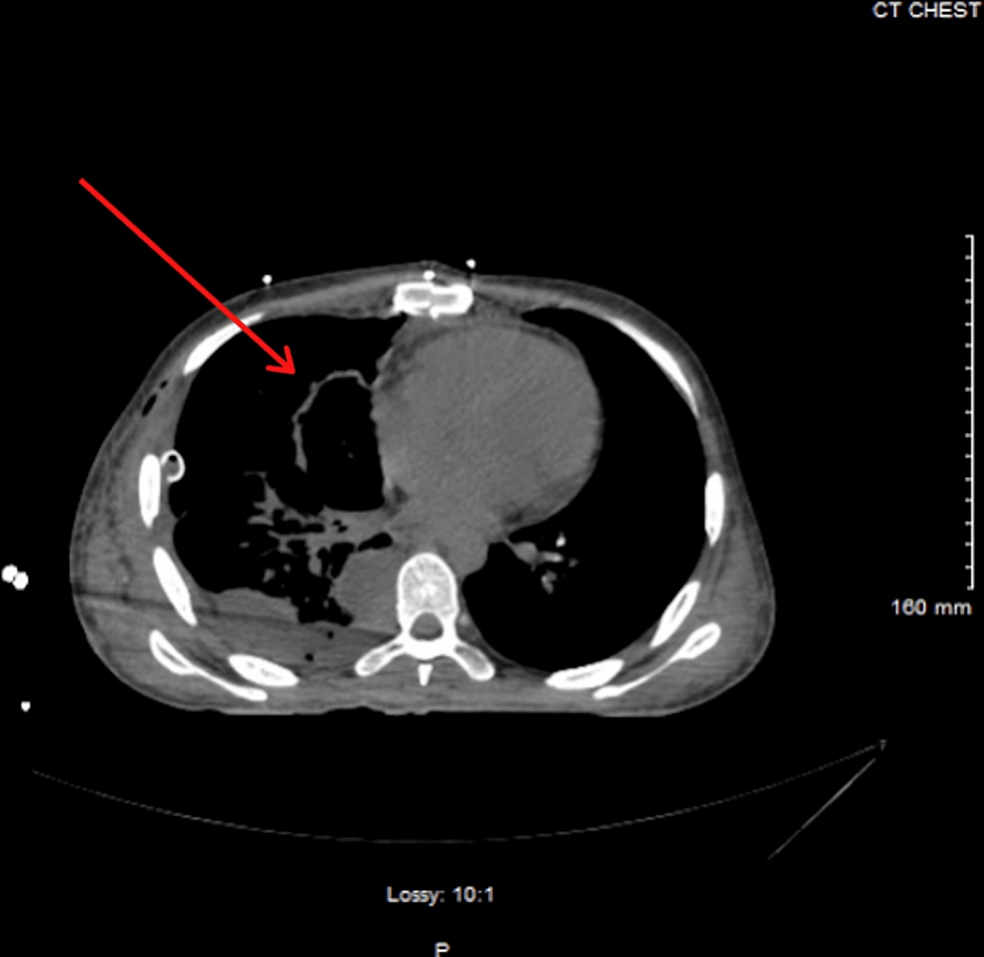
Axial section of a chest computed tomography without intravenous contrast showing interval resection of the mediastinal mass post-surgically.
